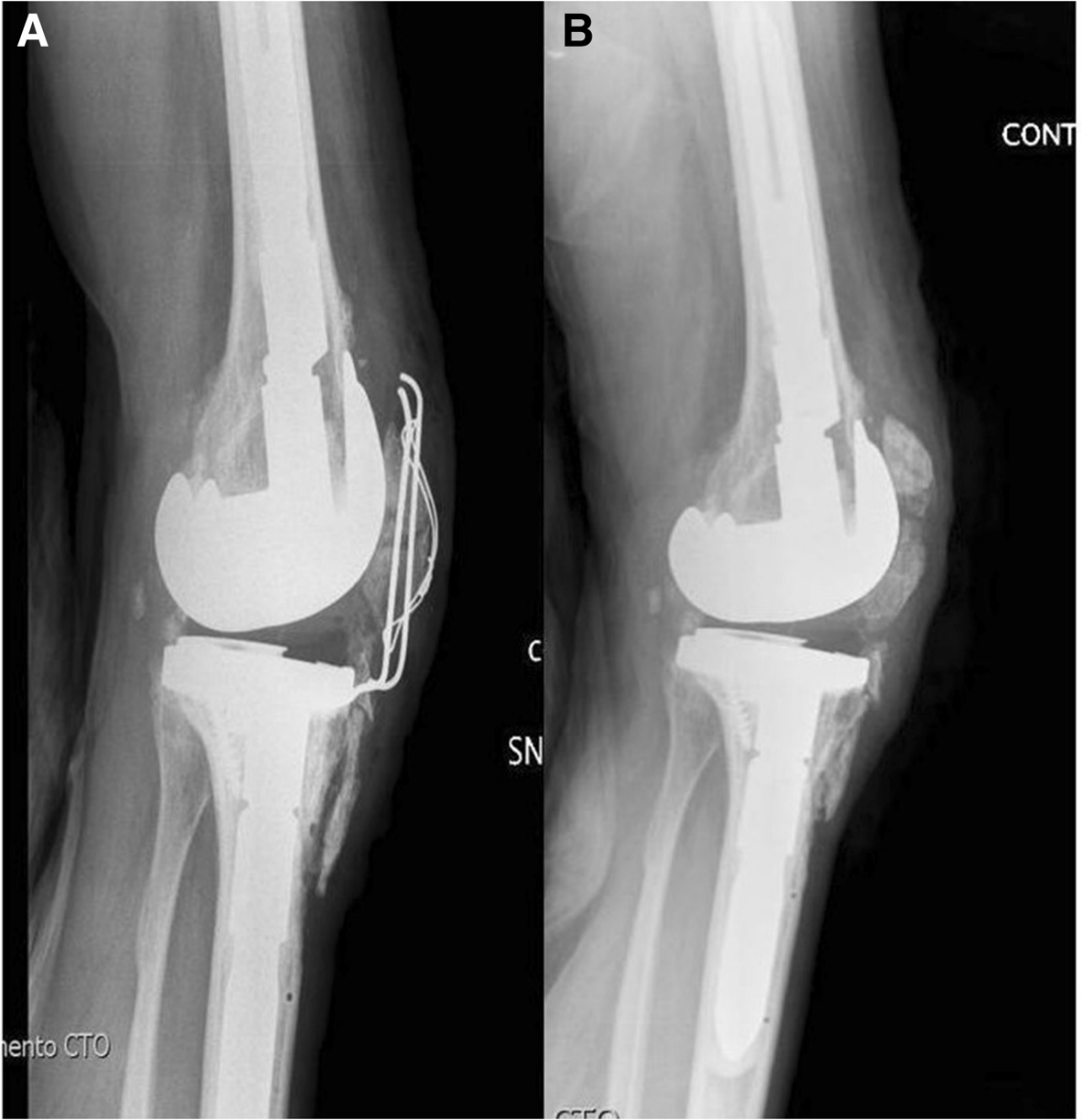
A-B: Open reduction and internal fixation of the patella fracture and tibial tubercle pseudarthrosis and 36 months follow-up radiographs, showing healing of the tibial tubercle and incomplete patella fracture healing.
